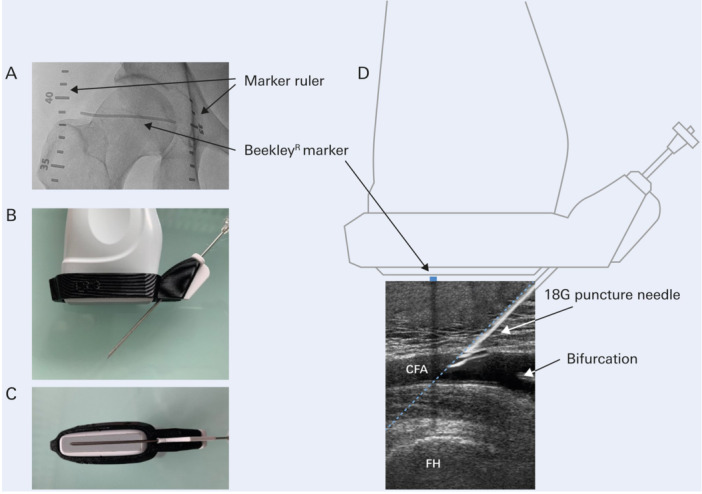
Ultrasound‐guided in‐plane puncture as used in the PARFEM trial. An ultrasound guided in‐plane puncture technique was used in which the CFA and the femoral head (FH) were visualized in the longitudinal plane (D). The center of the FH served as the upper border, and the bifurcation of the CFA as the lower border of the targeted puncture height. Within this zone, a suitable site for vessel puncture was identified. To ensure that the needle was continuously visualized in the displayed ultrasonic plane, a needle guide was used (B, C). Utilizing a displayed 45‐° reference line (D), the needle was advanced under ultrasound guidance. As an additional orientation landmark, a Beekley marker was placed horizontally at the level of the center of the FH with the aid of two rulers (A). The marker created a characteristic linear acoustic shadow (D), which helped to select the correct puncture height and to avoid “high sticks.” CFA = common femoral artery; FH = femoral head. [Color figure can be viewed at wileyonlinelibrary.com]
